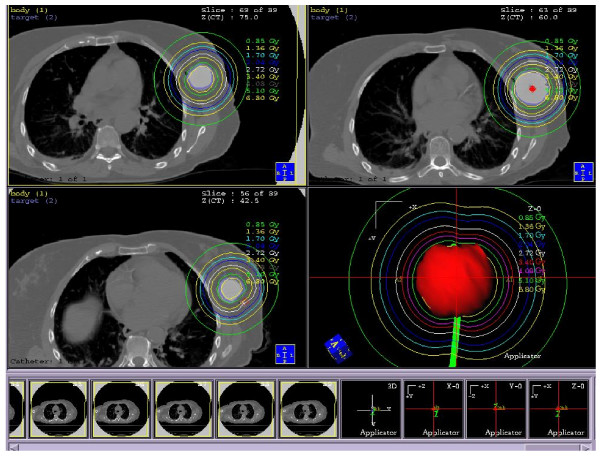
Axial CT images demonstrating the isodose pattern of the MammoSite balloon and surrounding critical normal tissues.
